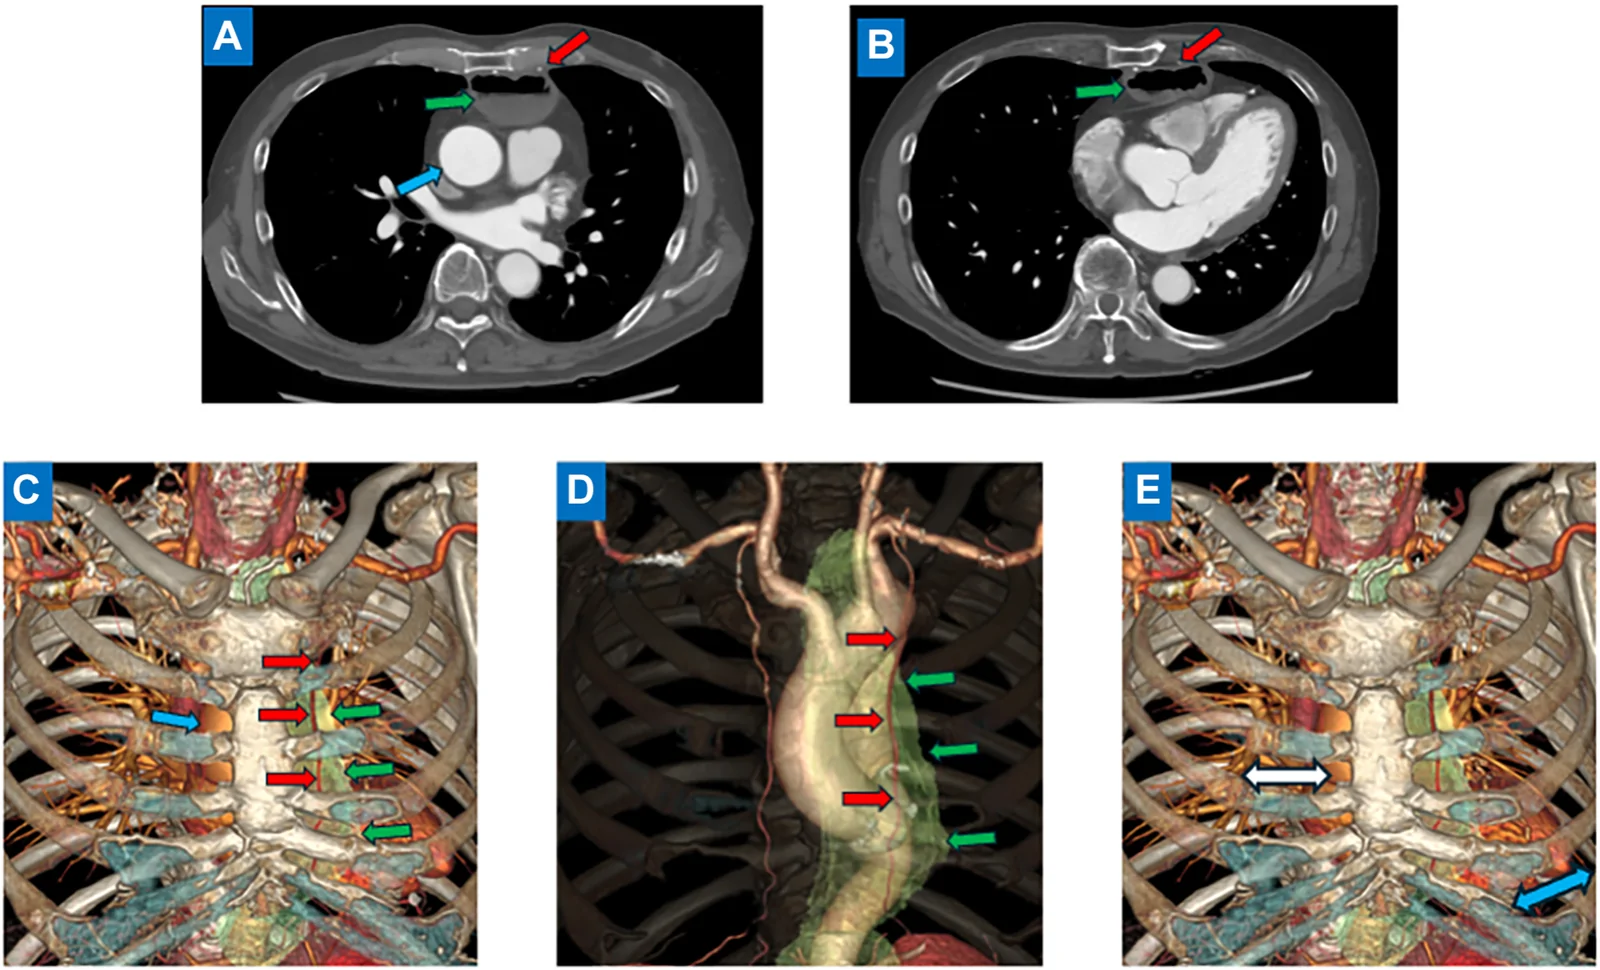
Preoperative computed tomography. (A) Axial image at the second intercostal level. A markedly dilated gastric tube lies directly behind the sternum (green arrow) in close contact with the left internal mammary artery (red arrow), and the ascending aorta (blue arrow) is positioned slightly rightward relative to the gastric tube. (B) Axial image at the fifth intercostal level. A markedly dilated gastric tube lies directly behind the sternum (green arrow) in close contact with the left internal mammary artery (red arrow). (C) A 3-dimensional computed tomographic reconstruction with the rib cage. The spatial relationships among the gastric tube, the left internal mammary artery, and the rightward-shifted ascending aorta correspond to those shown in (A, arrows). (D) A 3-dimensional computed tomographic reconstruction without the rib cage demonstrates the same anatomic configuration as (A, arrows). (E) A 3-dimensional computed tomographic reconstruction with the rib cage. The right minithoracotomy at the third intercostal space (white double-headed arrow) and the left minithoracotomy at the fifth intercostal space (blue double-headed arrow) are indicated.
